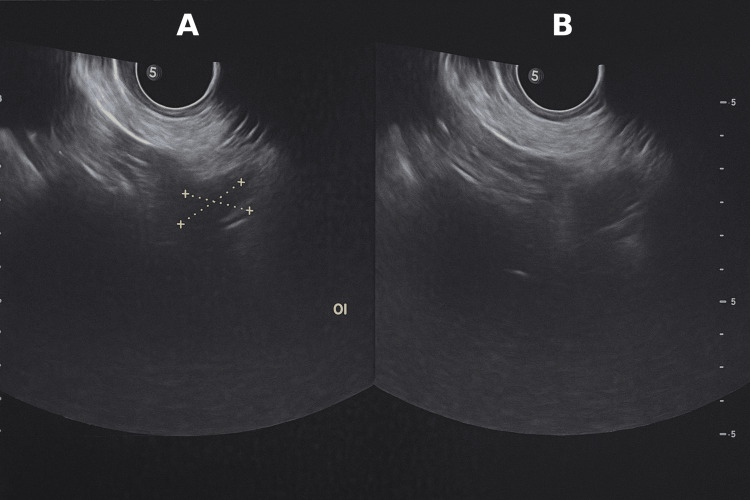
Transvaginal ultrasound, adnexal evaluation (A) Left ovary measured at 1.8 × 1.6 cm (yellow caliper marks), with preserved ovarian morphology. (B) Nonvisualization of the right ovary, consistent with prior right oophorectomy (depth scale at right margin).
